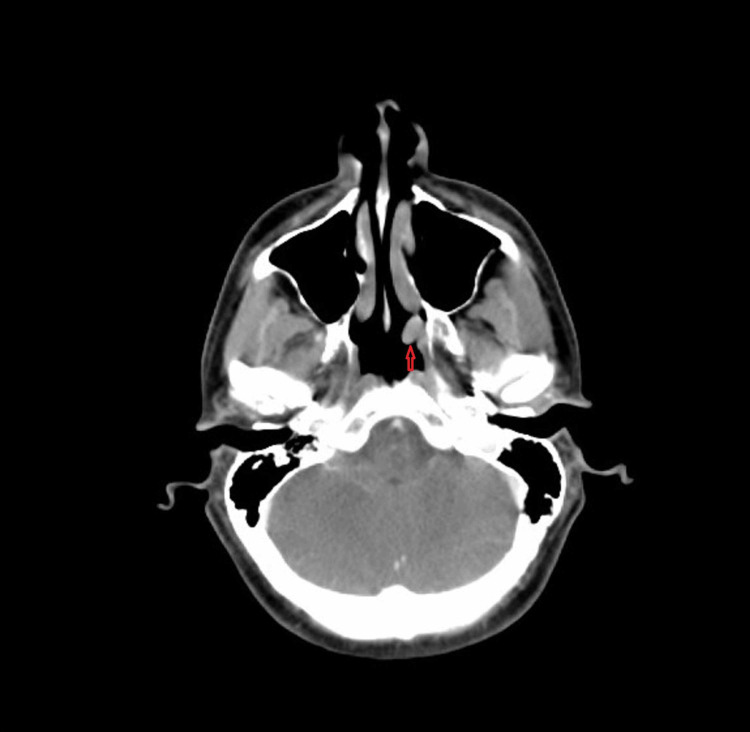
CT image showing soft tissue lesion located at the lateral wall of the left nasal cavity (red arrow)
